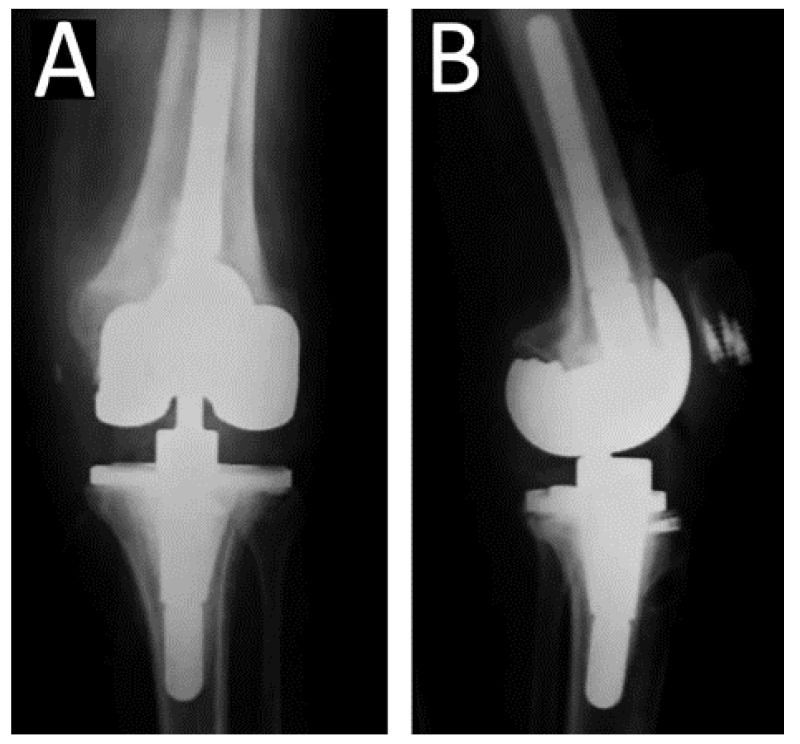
Due to persistent pain and stiffness the patient underwent revision total knee Arthroplasty (CCK) 6 years postoperatively. Due to intraoperative rupture of the patellar tendon, the patellar tendon was augmented with a semitendonous autograft, combined with a posterior tibialis tendon allograft and a synthetic graft jacket. The patellar tendon was sutured with bone anchors at the distal part of the patella. (A) Anteroposterior and (B) Lateral radiograph of the knee.
